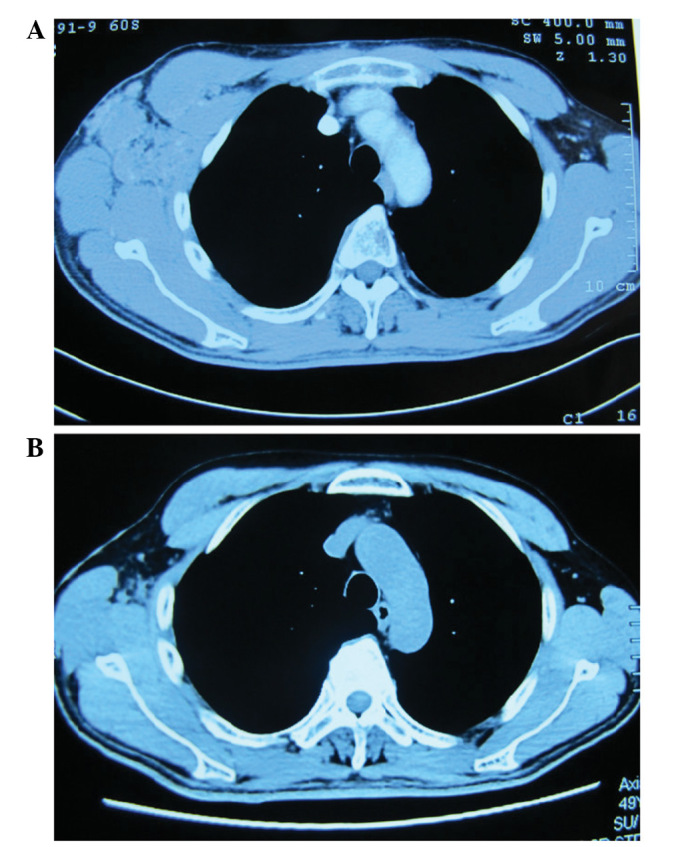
Computed tomography image of the complete right axillary neoplasm with surrounding tissues (A) prior to and (B) following therapy. The images reveal that the tumor reduced in size following 4 cycles of chemotherapy with paclitaxel and cisplatin.
